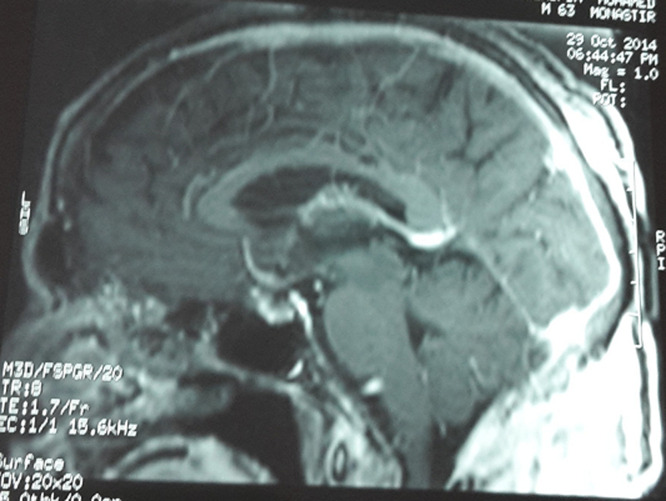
MRI showing superior sagittal sinus thrombosis
